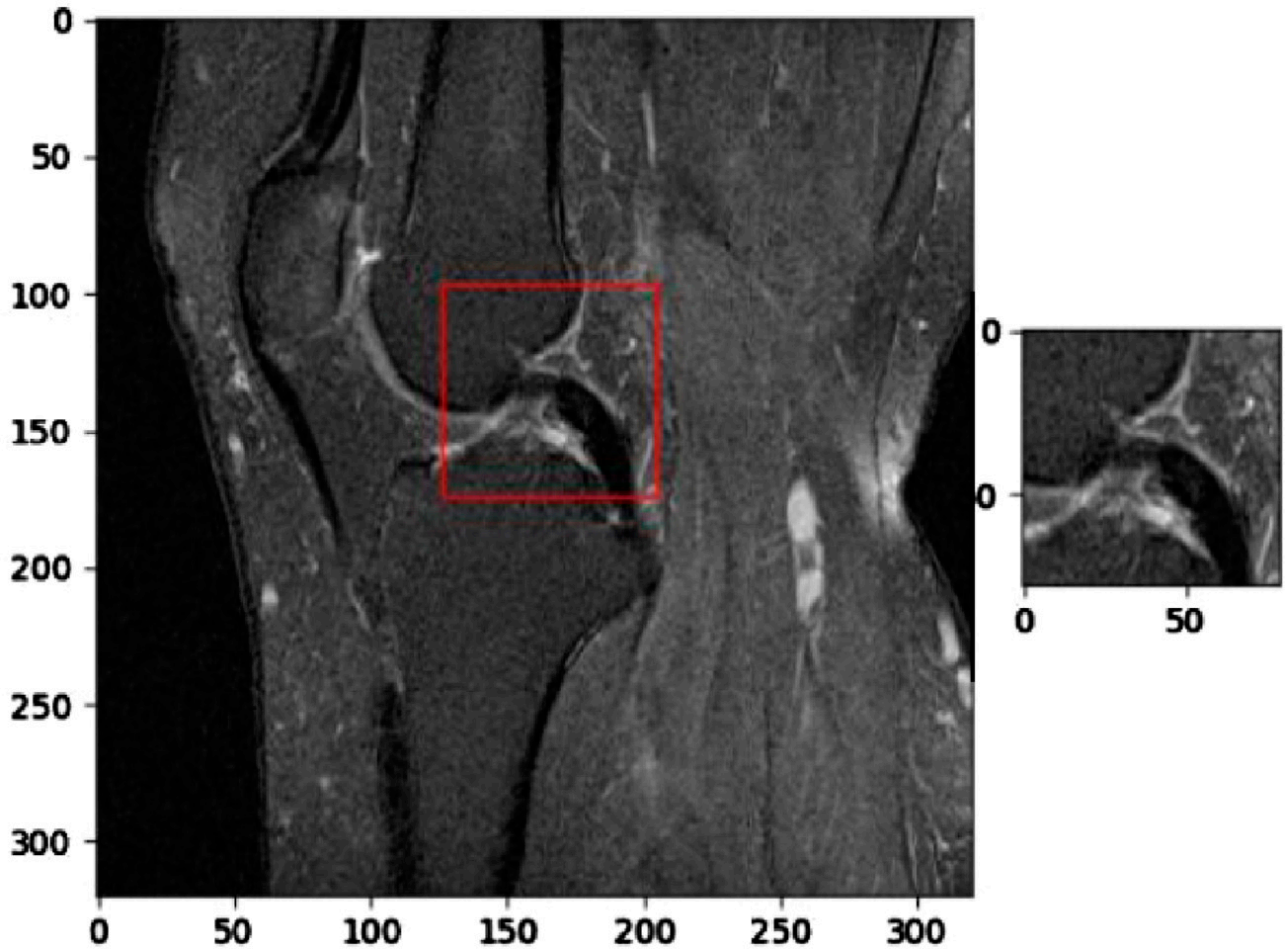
Segmented MRI image highlighting a complete anterior cruciate ligament (ACL) tear, a common and potentially debilitating knee injury that can be accurately detected using KneeXNet.
